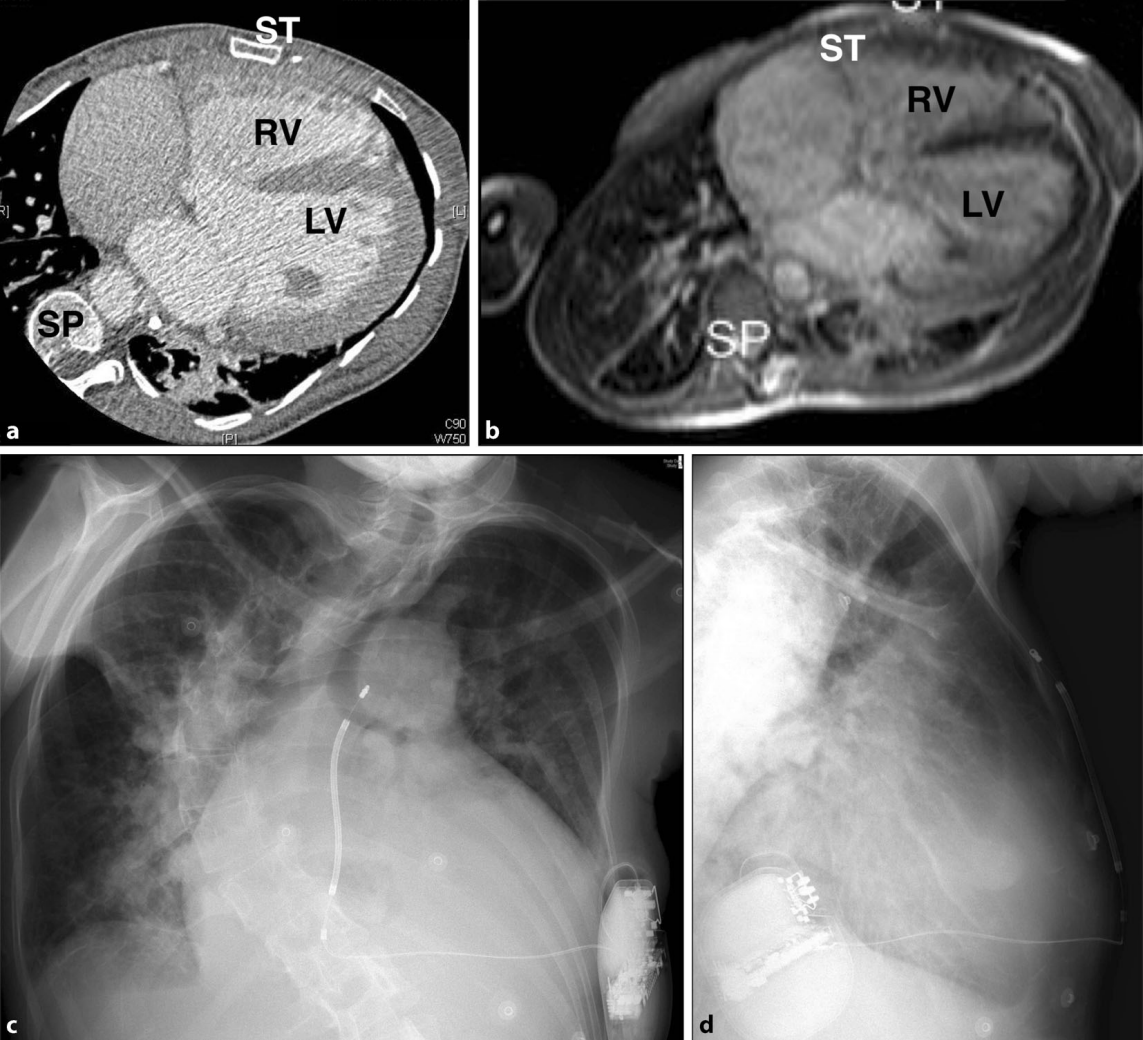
Patient with severe scoliosis, pulmonary atresia, ventricular septal defect and major aortopulmonary collateral arteries. a Computed tomography and b Balanced steady state free precession (b‑SSFP) magnetic resonance image. c  Anteroposterior and d  lateral radiographs demonstrating subcutaneous implantable cardioverter-defibrillator with excellent shock vector (subcutaneous coil has been placed to the right of the sternum, *RV* right ventricle, *LV* left ventricle, *SP* spine, *ST* sternum)
